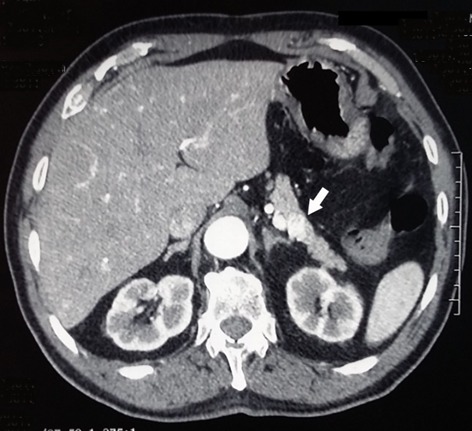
Abdominal CT scan showed a hypervascular lesion of 20mm localized in the tail of the pancreas
